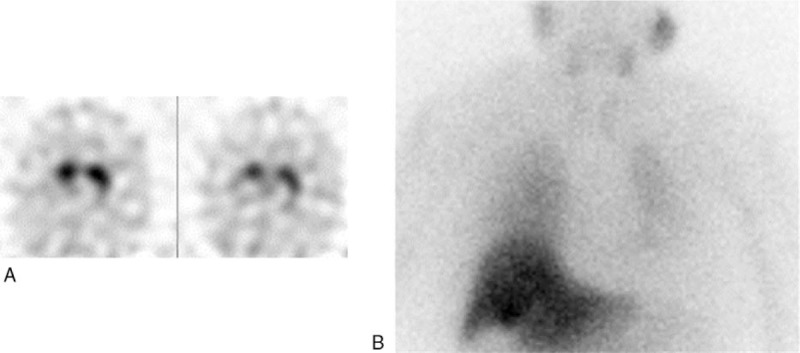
A 78-year-old female patient with uncertain parkinsonism and vascular lesions in subcortical areas, semioval centers, basal ganglia, and left temporal lobe at MRI. ^123^I-Ioflupane SPECT was pathological in both putamen nuclei (A) with also reduced BP values (1.7 and 1.8 in right and left putamen, respectively); ^123^I-MIBG cardiac scintigraphy (B) was pathological with reduced H/M values both in early (1.2) and delayed (1.10) phases. The patient was finally classified as PD. MRI= magnetic resonance imaging, SPECT = single photon emission computed tomography.
